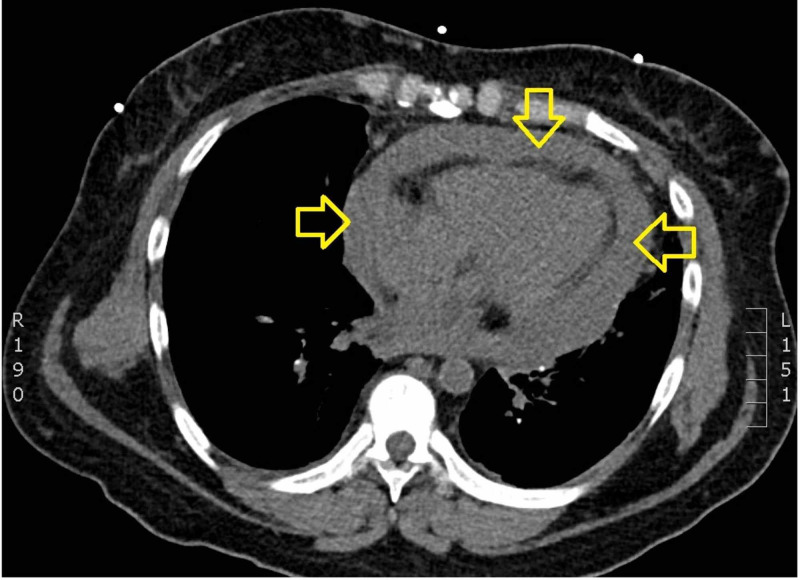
CT showing large pericardial effusion
